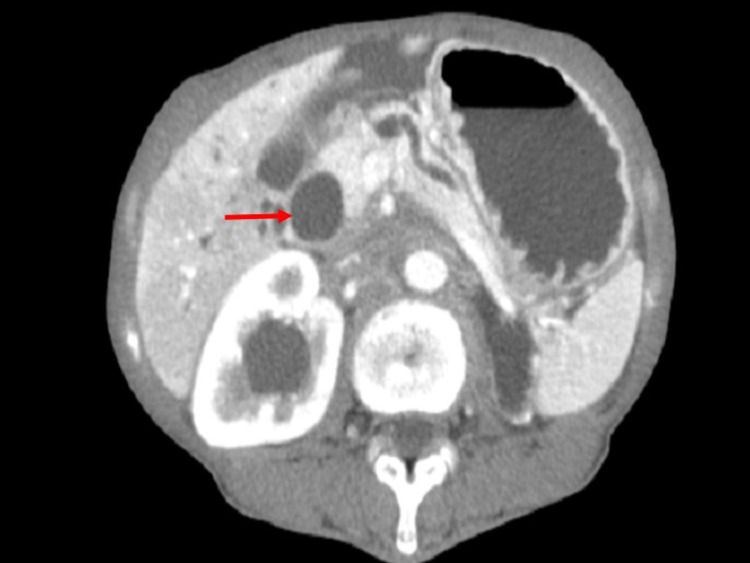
CT scan of the abdomen and pelvis showing distended common bile duct with dilation of the Wirsung duct
